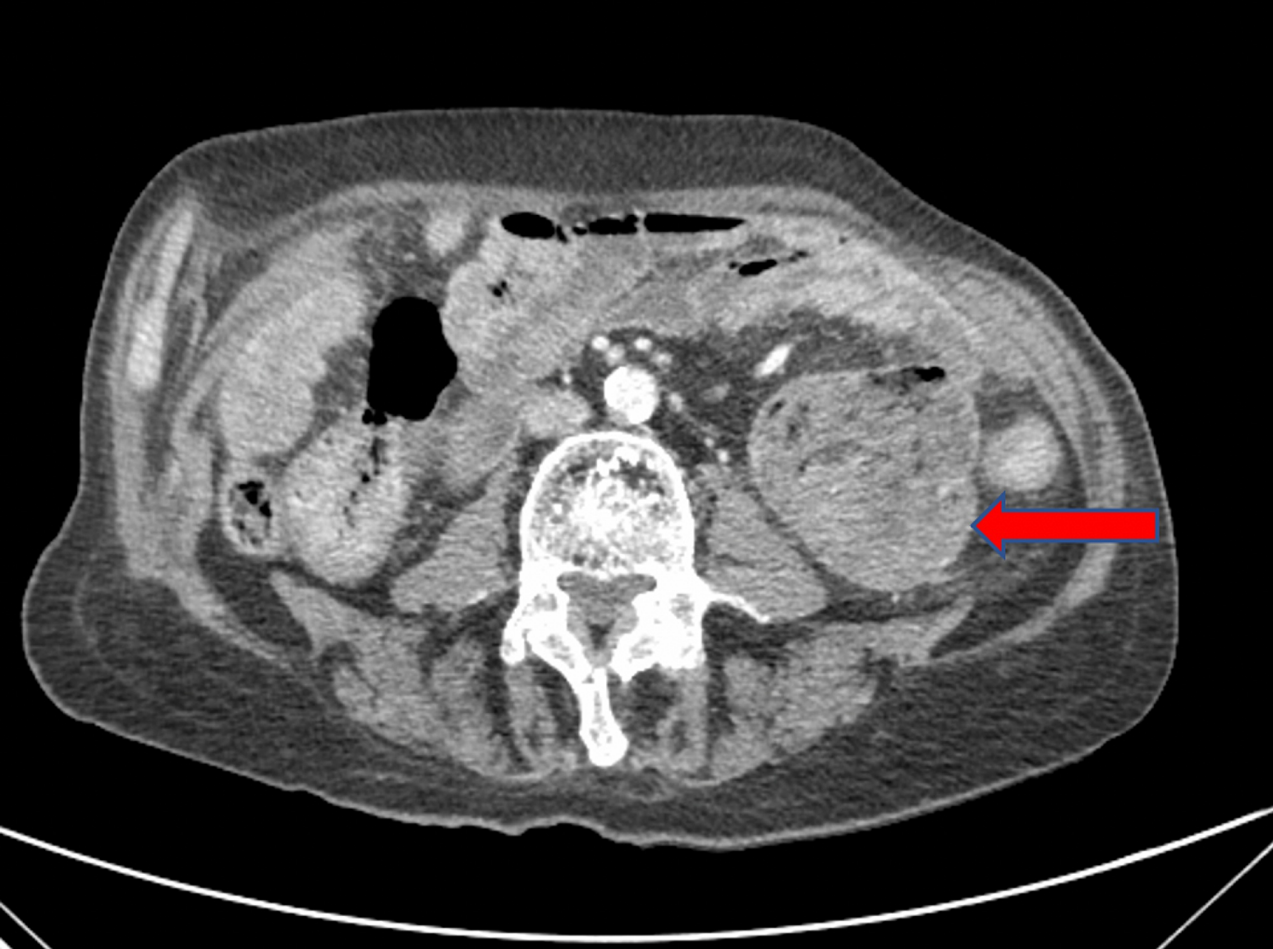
CT of the abdomen and pelvis. Red arrow: proximal jejunum is dilated up to 6.8 cm in diameter.
